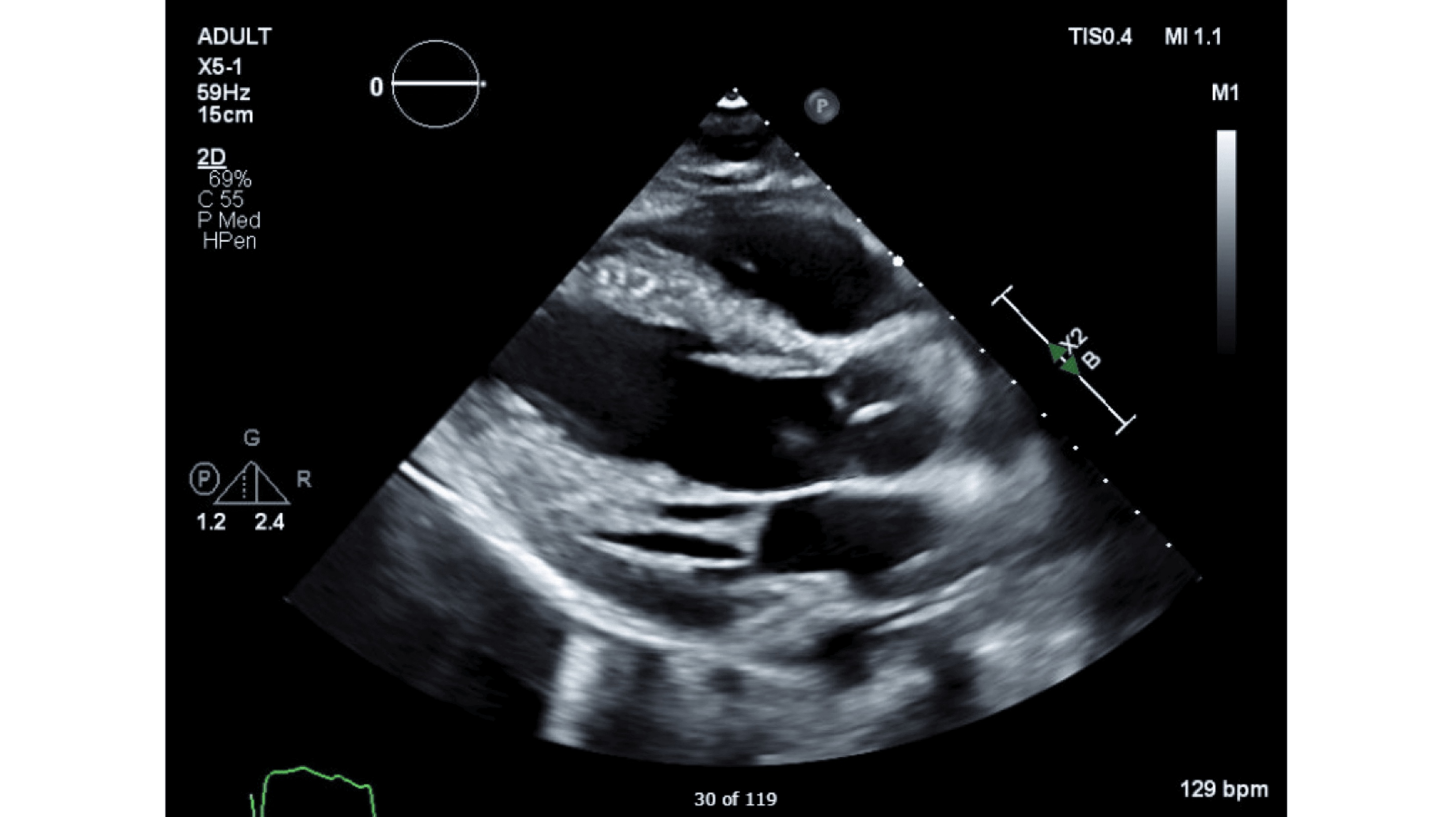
Parasternal long axis view of transthoracic echocardiogram done at presentation showing left ventricular ejection fraction of 20%.
